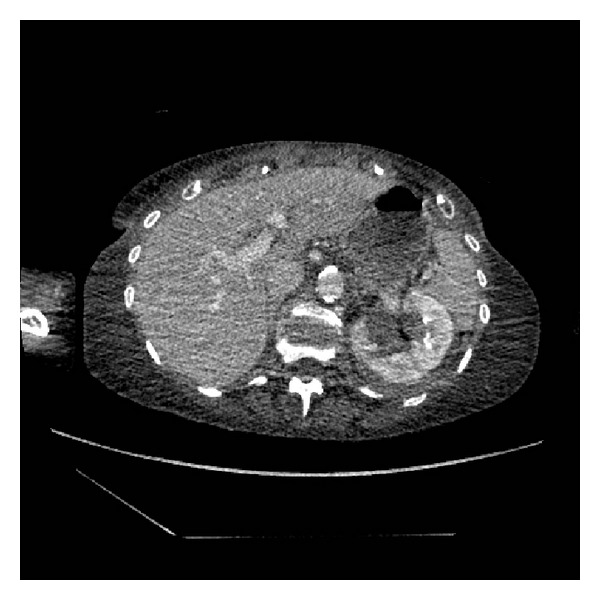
Split bolus contrast CT KUB: transverse section, left kidney.
